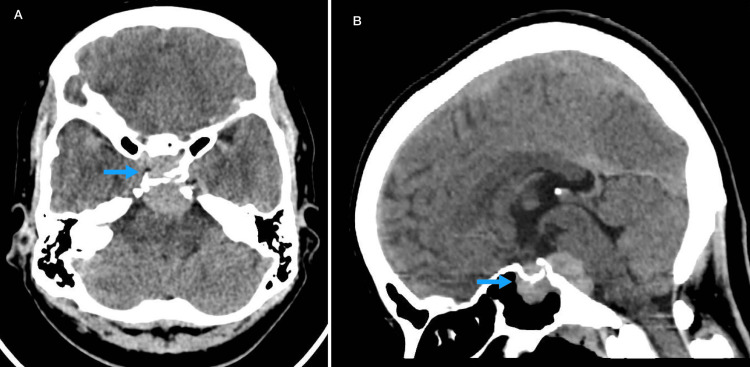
Computed tomography (CT) findings. (A) Axial non-contrast CT brain showing a hyperdense extra-axial mass arising from the sphenoid sinus (arrow). (B) Sagittal non-contrast CT brain showing the same hyperdense extra-axial mass arising from the sphenoid sinus (arrow).
